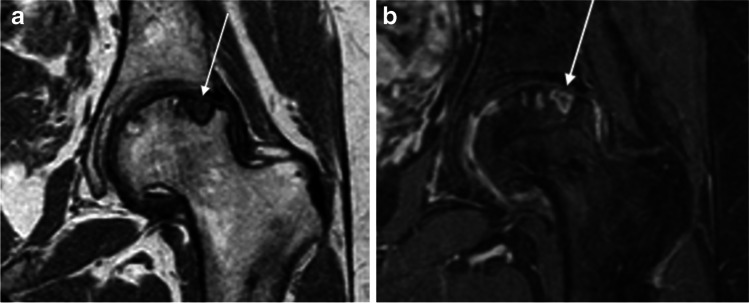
Left-hip MRI of active erosion in an 18-year-old woman with juvenile idiopathic arthritis (JIA). a, b Coronal three-dimensional (3-D) T1-weighted turbo spin-echo (a) and post-contrast 3-D gradient echo with fat saturation (b). These images show an active erosion (erosion filled with enhancing pannus) (*arrows*)
